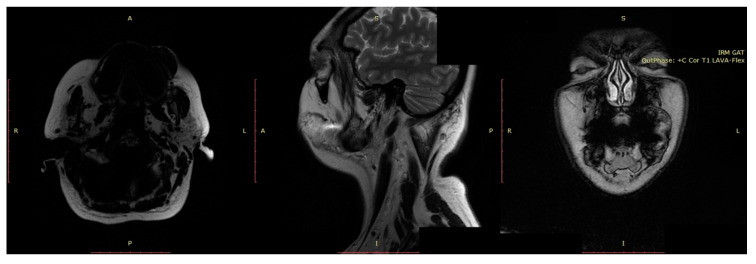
MRI of a patient with an AVM at the level of the upper lip and left aspect of the face, R—right, L—left, A—anterior, P—posterior, S—superior, I—inferior.
